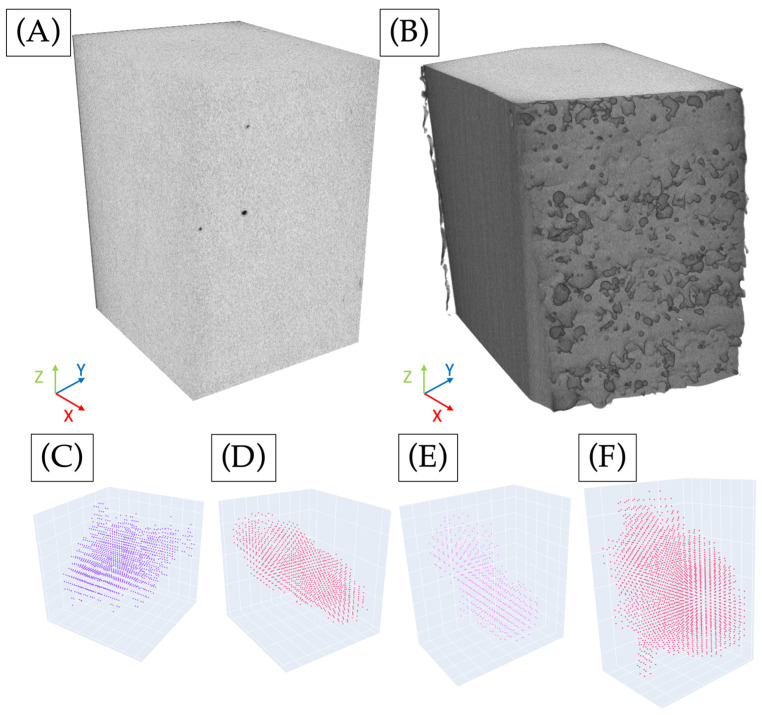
Sample X-ray CT output cross-section of a parallelepiped core. (A) Segmented image, (B) original image, and examples of pore volumes: (C) 1474 µm^3^, (D) 1236 µm^3^, (E) 583 µm^3^, and (F) 2285 µm^3^.
